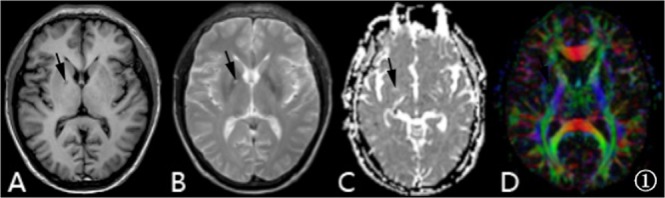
A 42-year-old male patient with CMS, Hb 220 g/l. Images (A–D) are T1-weighted, T2-weighted, ADC and FA colour maps, respectively. The black arrow points to the intracranial haemorrhage foci in the right basal ganglia region.
